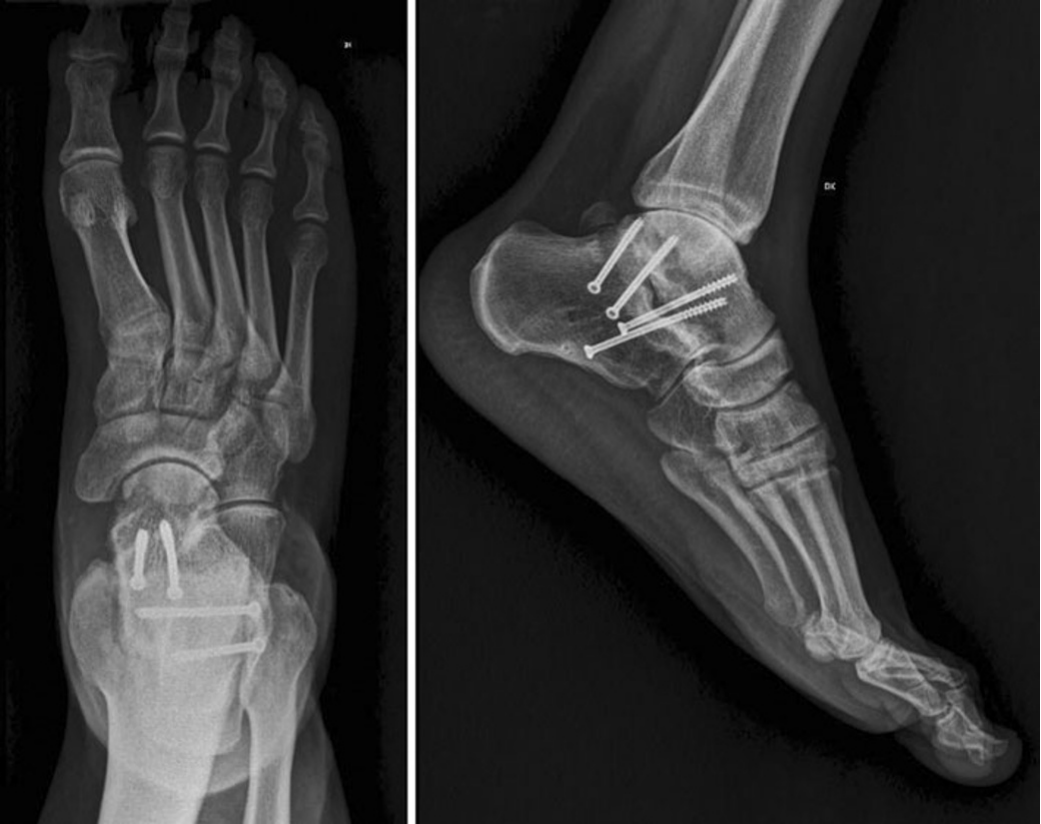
Arthrodesis with two screws in the anterior subtalar joint and in the posterior subtalar joint. Anteroposterior and lateral X-ray projections after 2 years of follow-up. No signs of avascular necrosis are observed
